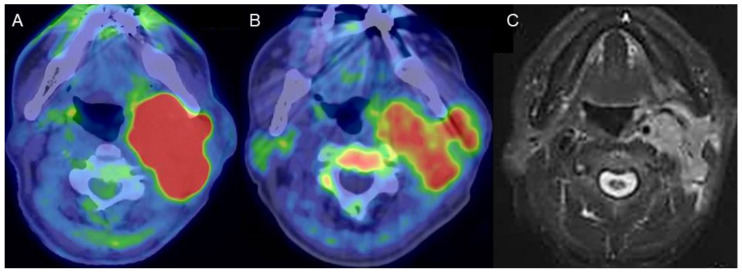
A man in his 80s with carcinoma arising in the left parotid gland. (A) Fused FDG-PET/CT image (axial view), (B) fused 4DST PET/CT image (axial view), and (C) gadolinium (Gd)-enhanced MRI (axial view). Gd-enhanced MRI shows homogeneous enhancement of an irregular-shaped lesion in the left parotid gland. High FDG uptake (SUV_max_: 21.8) as well as lower, but unequivocal, 4DST uptake (SUV_max_: 6.0) were confirmed in the lesion.
